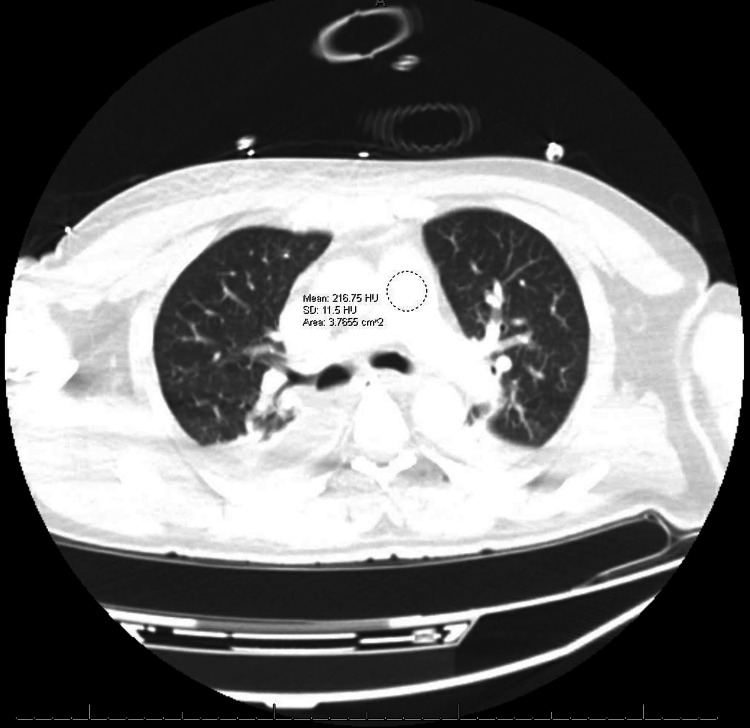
CT scan performed one day postoperatively demonstrating bilateral infiltrates
